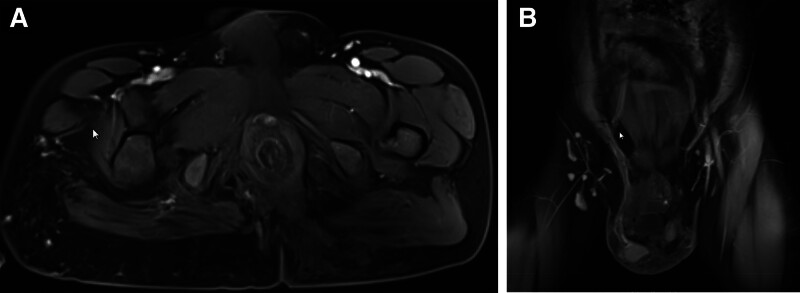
Pelvic MRI revealed rectal wall thickening, mesenteric infiltration, and swelling, along with visible lymphatic dilation. Varicose veins were also noted in the sacral anterior, scrotal region, and both buttocks. Within the scanning range, there was atrophy of the thigh muscles and the muscles in the right buttock, including gluteus maximus and gluteus minimus. MRI = magnetic resonance imaging.
